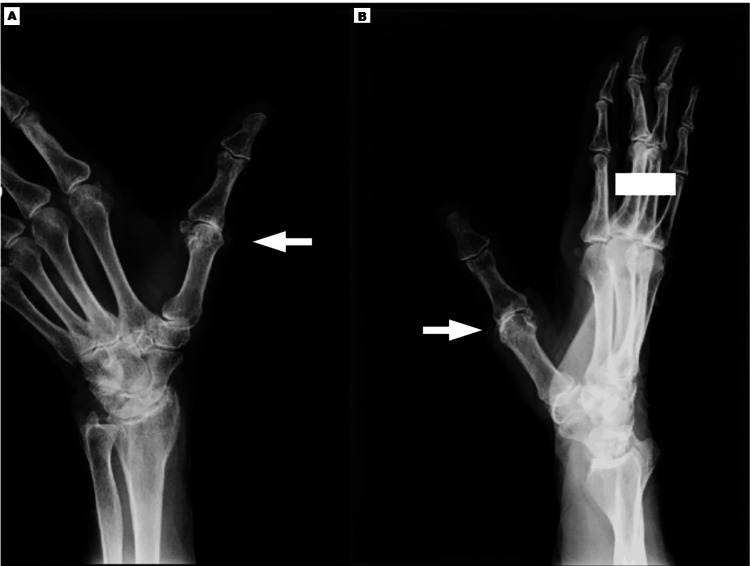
Radiographic Evaluation of First Metacarpophalangeal Joint Degeneration for Case 1 (A, B) Radiographic evaluation reveals advanced degeneration of the first metacarpophalangeal joint, consistent with Eaton-Littler stage III osteoarthritis.
